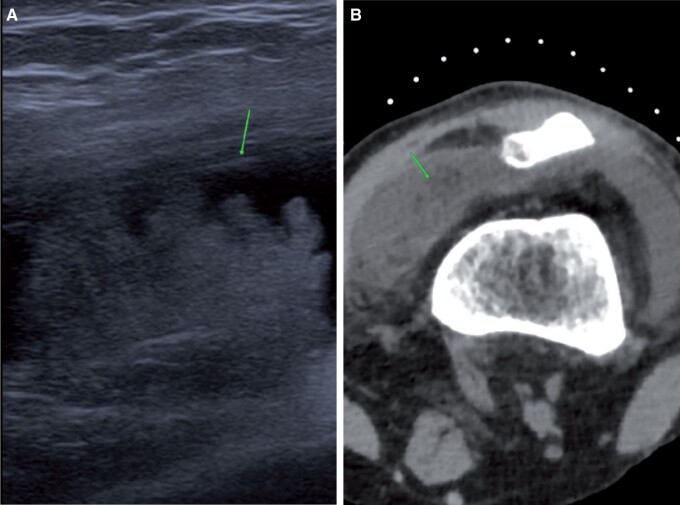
Lipoma arborescence: (A) ultrasound image showing echogenic material (arrow) in the suprapatellar recess. (B) Corresponding planning CT image showing macroscopic fat (arrow) in the supra patellar recess.
